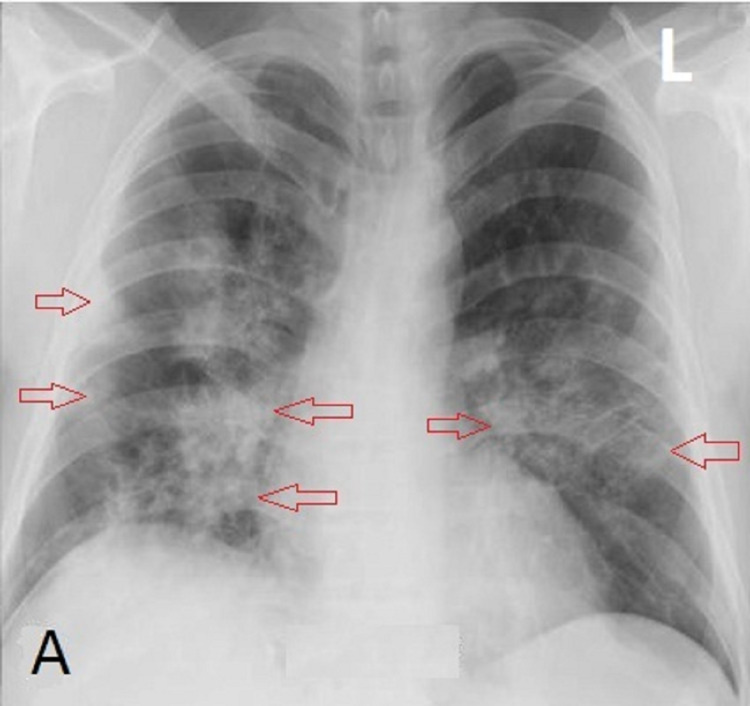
During admission, chest X-ray (A) showed bilateral ground-glass opacities (red arrows).
